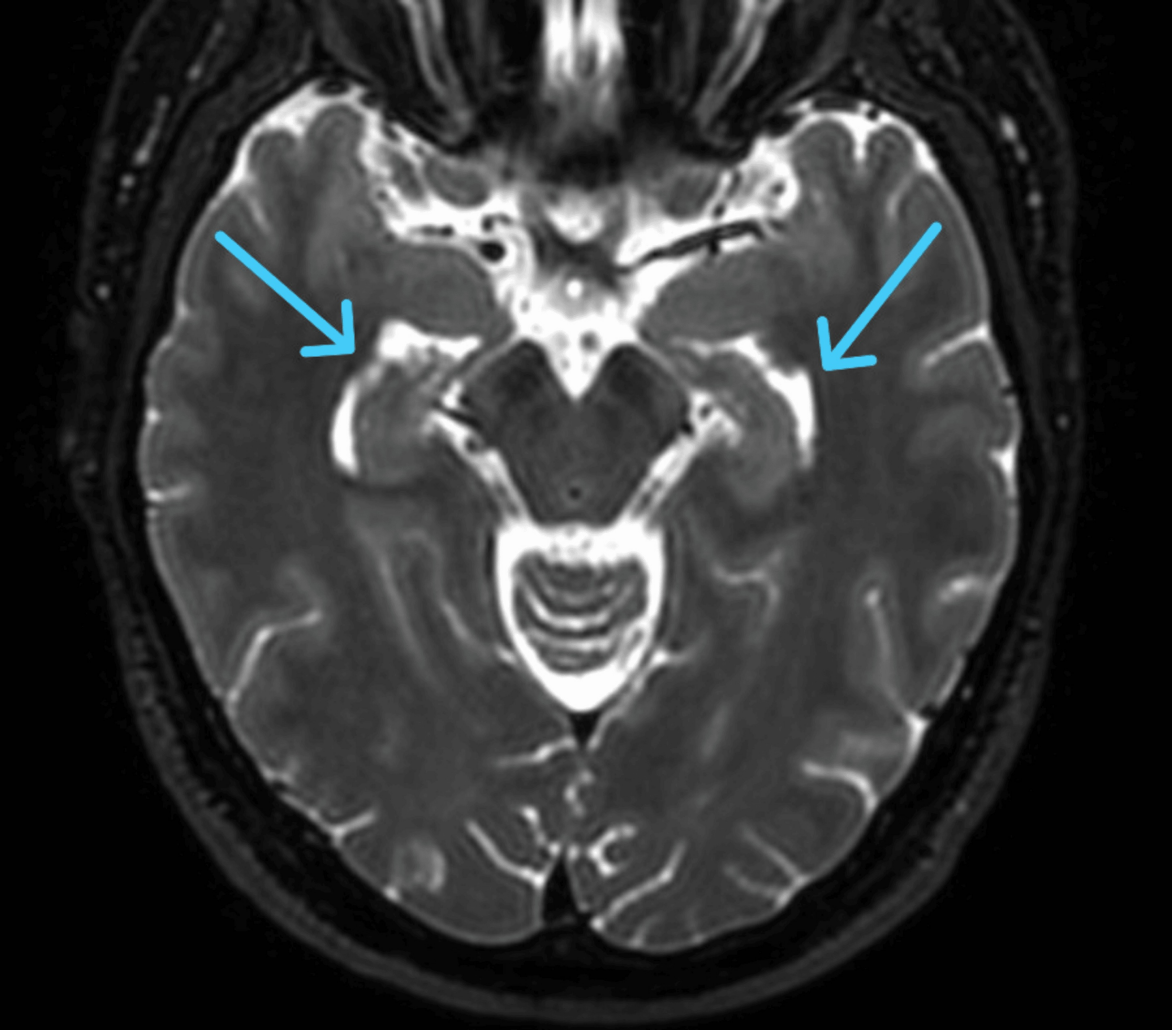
Brain MRI showing calcifications in Fahr’s syndrome. Dense calcification is evident in the basal ganglia, with lesser involvement of the cerebellar dentate nuclei (blue arrows). These imaging findings are suggestive of Fahr’s disease/syndrome, reflecting abnormal calcium deposition in deep gray matter structures.
